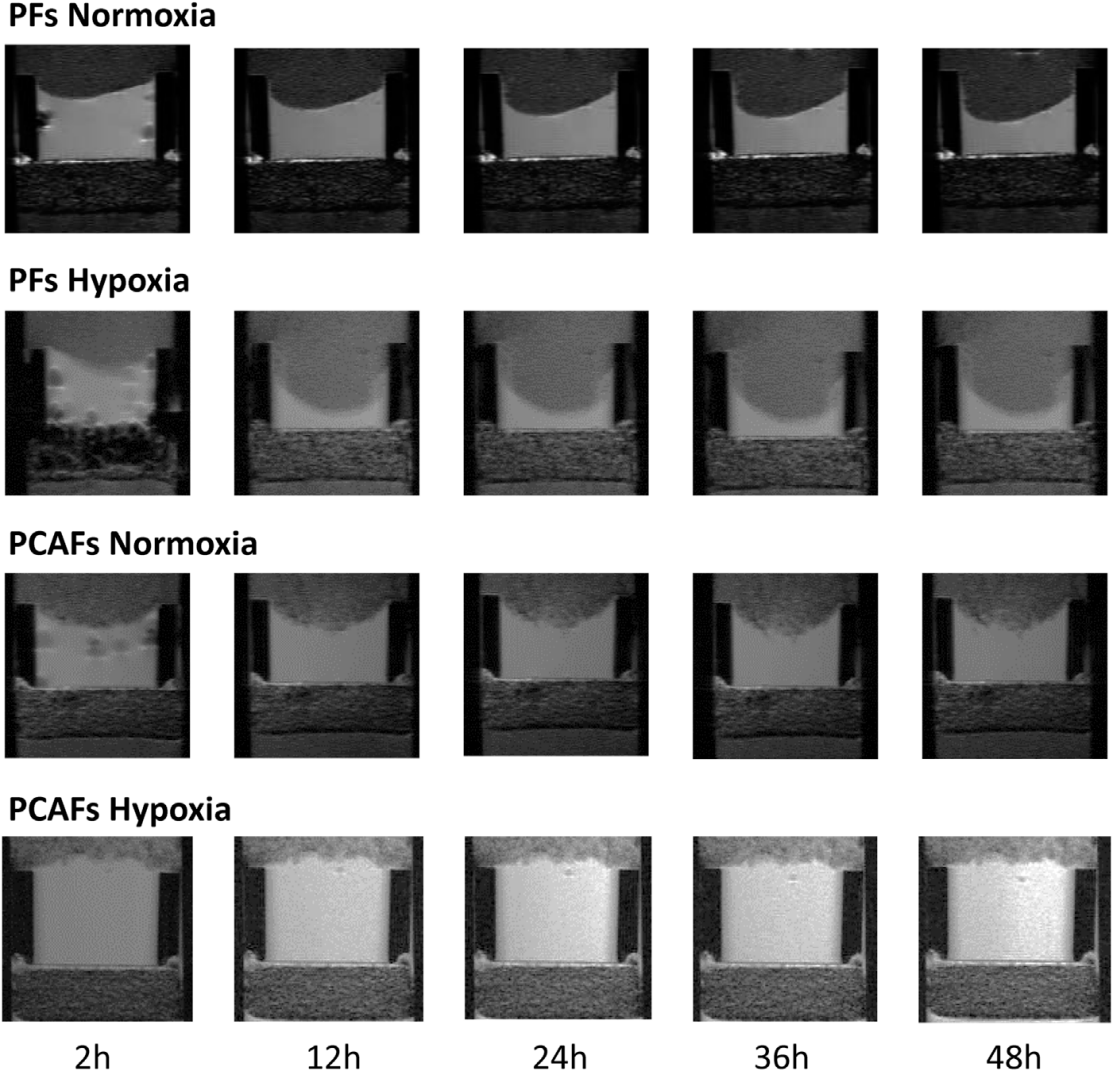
Representative T1-weighted ^1^H MR images displaying the region of the ECM chamber to demonstrate degradation of the ECM by PFs and PCAFs under normoxia and hypoxia over a period of 48 h.
